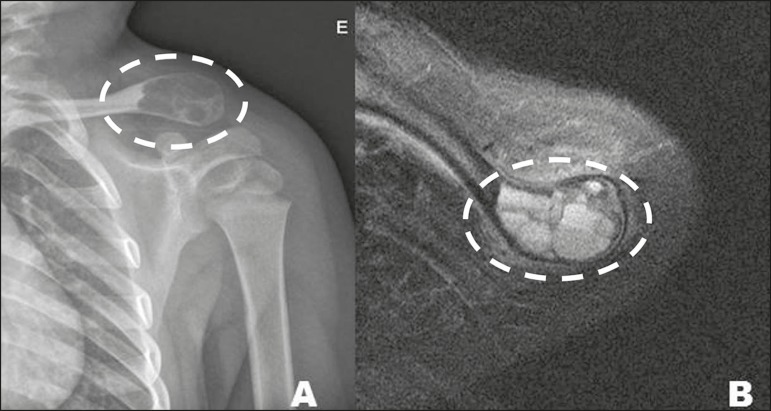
A 2-year-old male examined five days after a fall. An MRI scan shows
